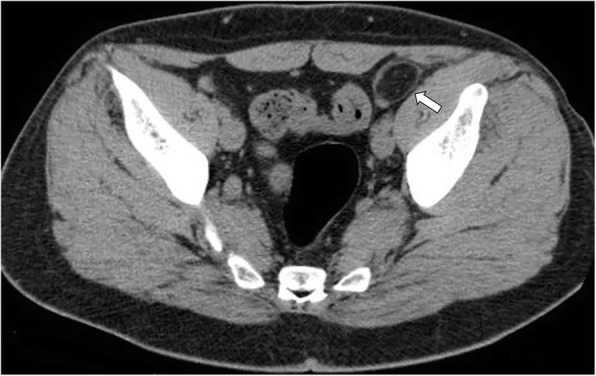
Acute epiploic appendagitis in a 43-year-old man with presumptive clinical diagnosis of colonic diverticulitis. Axial non-contrast CT image shows a pericolonic fat-density lesion (arrow) that abuts anteriorly the colon-sigmoid junction in the left iliac fossa
